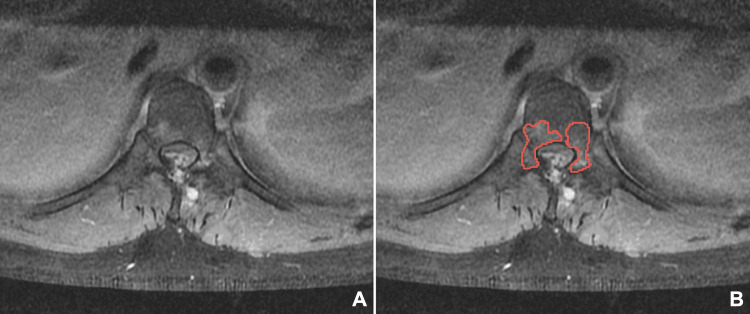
Axial T1 weighted contrast-enhanced MRI image demonstrating hyperintense bone marrow edema (red solid outline) within the vertebrae and spinal cord compression. The pathology is highlighted (B) as compared to (A).
